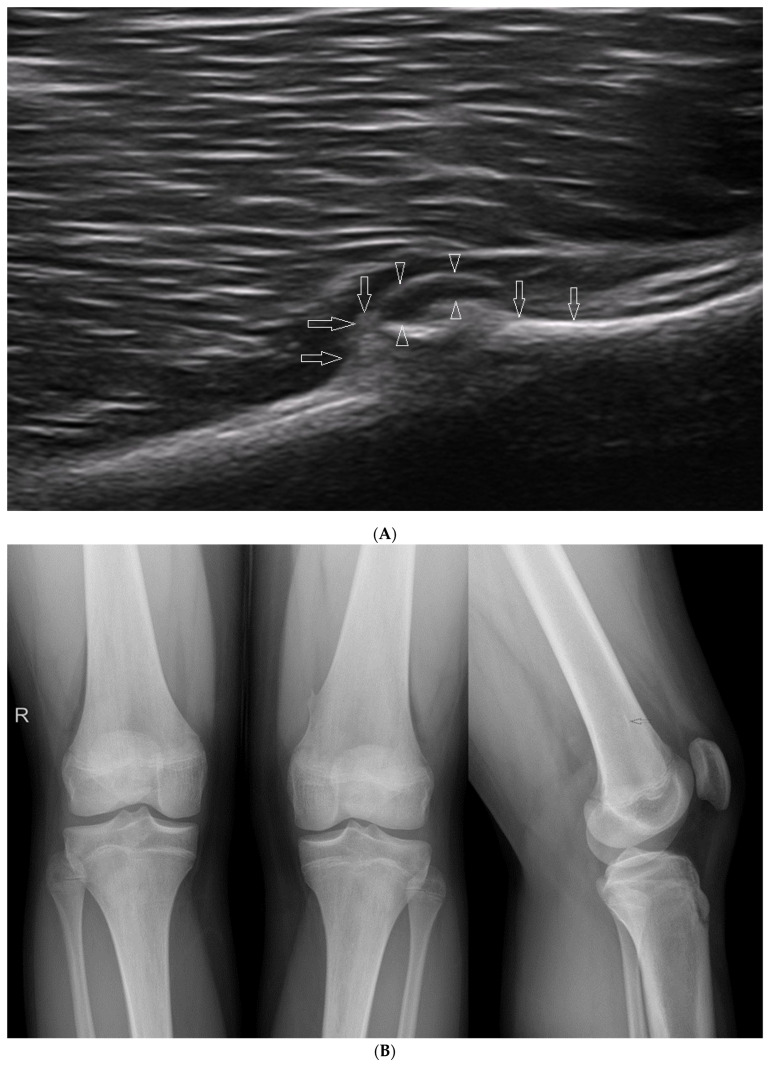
(A) An example of an osteochondroma exostosis of the left femur of a 16-year-old boy. Long axis view. Arrows—bony part of the exostosis; arrowheads—partly built of hyaline cartilage (anechoic). Linear probe 3–19 MHz. (B) Comparative anteroposterior X-ray image of the knee joints from the same patient and lateral image of the left knee joint, with visible osteochondroma of the left femur. Small empty arrow—longitudinal shadow corresponding to the exostosis on the lateral radiograph.
